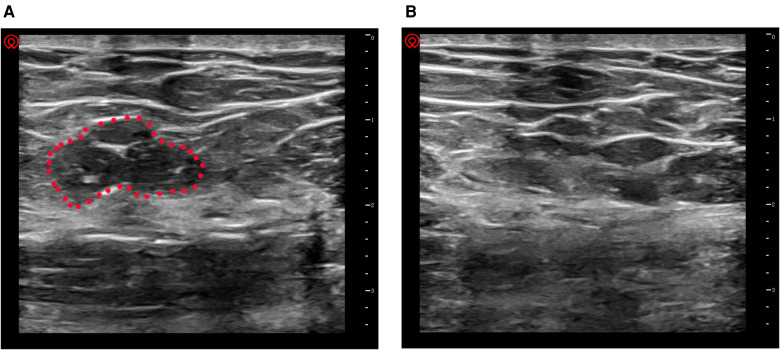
Verifying dead space by ultrasonography. (A) before incisional pressing. there was an obvious dead space under the incision (the area in the red dotted circle); (B) after incisional pressing, the dead space disappeared.
